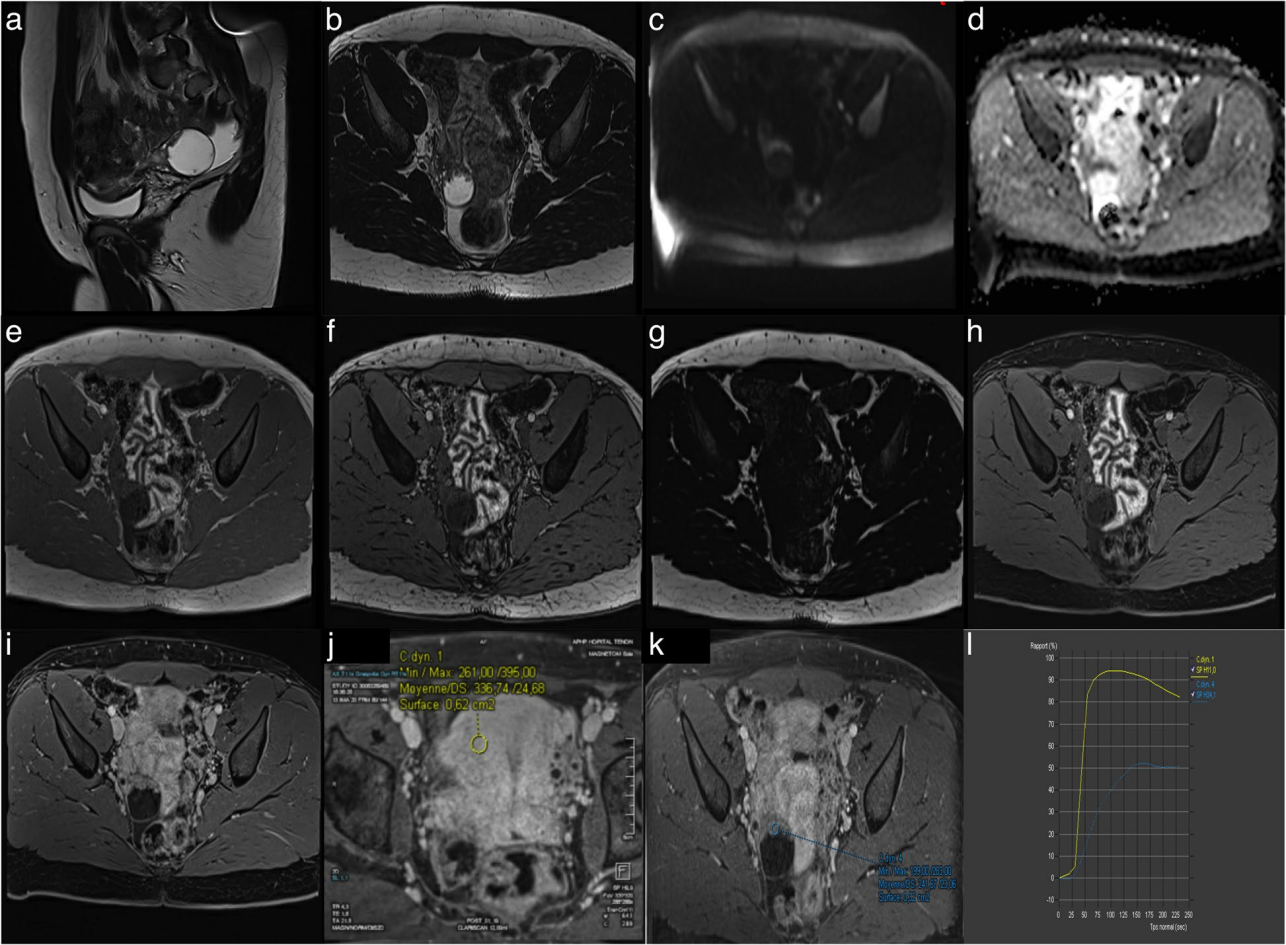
Optimal MRI protocol to perform O-RADS MRI Risk scoring. a Sagittal T2-weighted sequence without fat saturation. b Axial T2-weighted lombo-pelvic sequence. c Axial DWI lombo-pelvic scan (*b*: 1400 s/mm^2^). d Corresponding axial ADC map. e Axial unenhanced T1-weighted in-phase. f Axial unenhanced T1-weighted opposed-phase. g Axial unenhanced T1-weighted Fat sequence. h Axial unenhanced T1-weighted water sequence. i Axial late-gadolinium T1-weighted sequence. j, k DCE T1-weighted sequence with myometrial curve (yellow curve) and region of interest within the solid ovarian tissue (blue curve) (l). This was classifified as an O-RADS MRI 4, and hypothesized to represent a serous borderline cystadenoma
